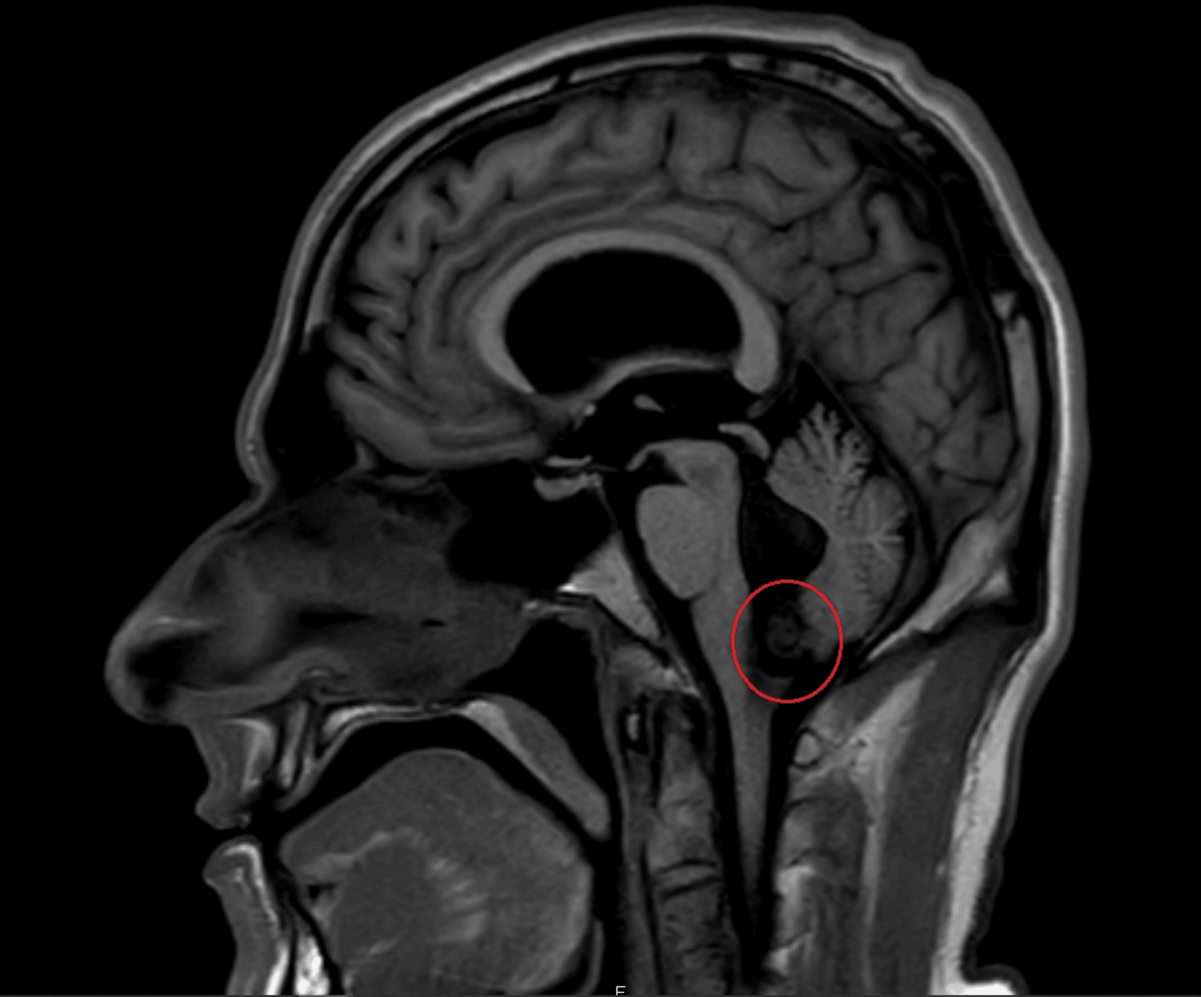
MRI head demonstrating a 1 cm intraventricular mass within the fourth ventricle at the foramen of Magendie resulting in moderate obstructive hydrocephalus (red circle).
